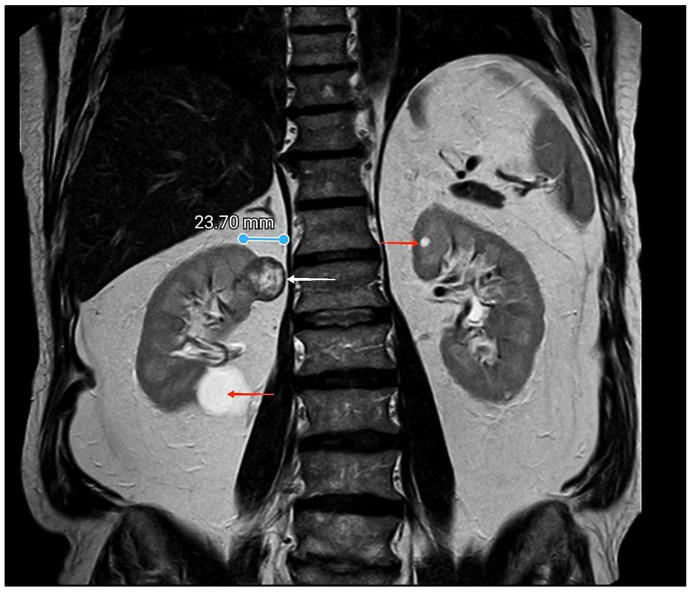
Magnetic resonance imaging (MRI) of the abdomen. A 66-year-old man presented to his urologist for a regular benign prostate hyperplasia work-up. A well-circumscribed and dominantly hyperechoic mass lesion with an approximate diameter of 25 mm was discovered incidentally in the upper pole of the right kidney on an abdominal ultrasound. The MRI scan verified the well-demarcated and solid lesion measuring 27 mm × 22 mm with increased signal intensity on T2-weighted sequences with respect to the kidney parenchyma (white arrow). A simple cyst with a diameter of 32 mm was described in the lower pole of the same and opposite kidney (red arrows). The complete blood count, comprehensive metabolic panel, and urinalysis were unremarkable. The patient had no malignancies in their past medical history. Partial nephrectomy was indicated. Intraoperatively, extensive adhesions to the peritoneum and the liver were identified. Due to the patient’s habitus, the superior pole of the kidney was in a more subhepatic position than presented by preoperative imaging. Also, adherent perinephric fat was determined. The presence of inseparable adipose tissues adhered to the kidney parenchyma, combined with the tumor location at the posterior side of the upper pole, made it impossible to provide an adequate plane to perform a partial nephrectomy. Taking that into consideration and to also decrease the possibility of excessive intraoperative bleeding, the decision was made to proceed with radical nephrectomy. The patient underwent radical nephrectomy without complications in the postoperative course.
